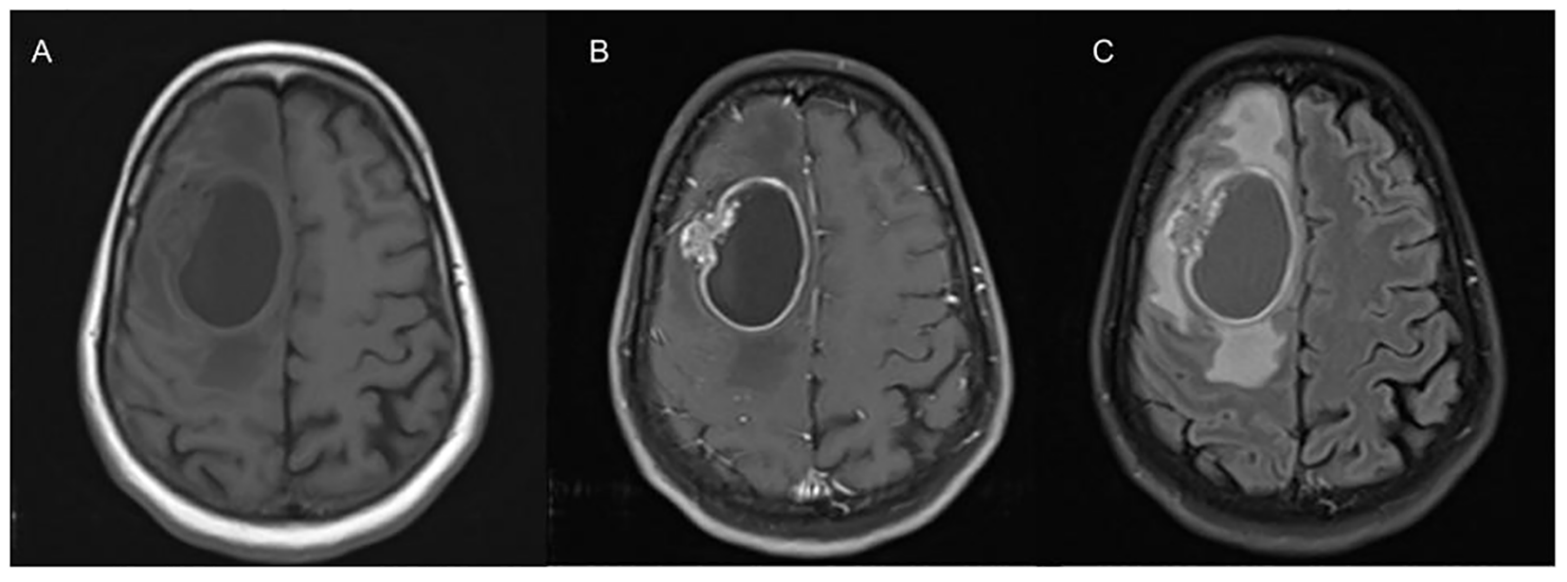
This is an axial view of T1 (A) revealing an ovoid-shaped hypodensity representing a cystic lesion in the right frontal lobe with perilesional edema with mass effect on contralateral hemisphere and posteriorly. On T1 post contrast imaging (B), there is hyperintensity representing enhancement in the margins with heterogenous hyperintensity on the lateral wall appearing to invade brain parenchyma. FLAIR imaging (C) showing significant perilesional edema causing effacement of the sulci.
